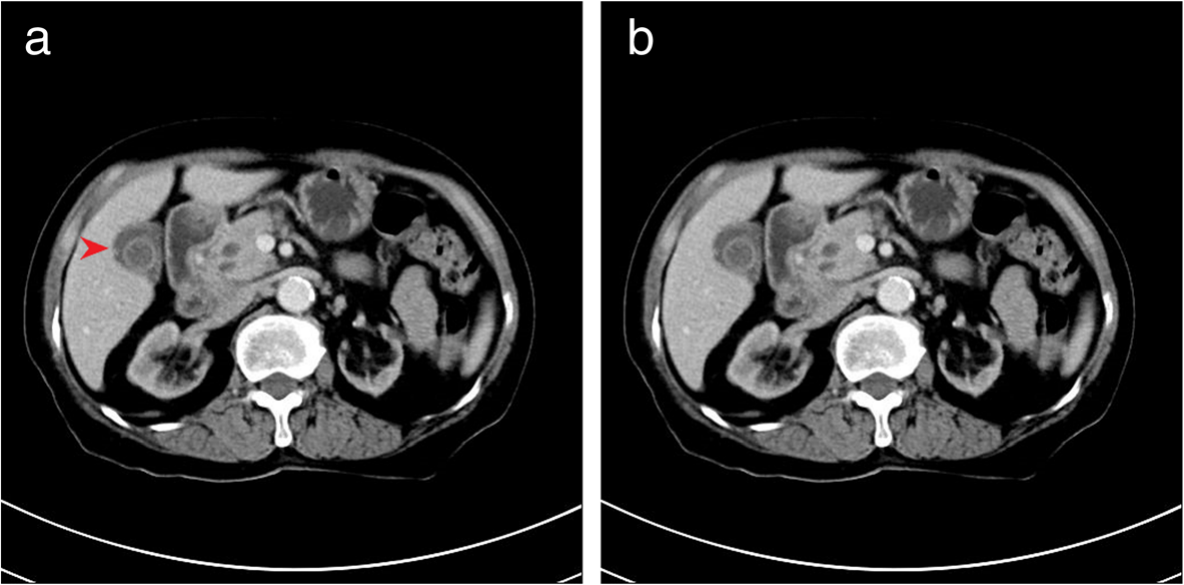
CT scan showing an increased gall bladder volume with a 1.7*1.8 cm irregular soft tissue density on the neck of the gallbladder
